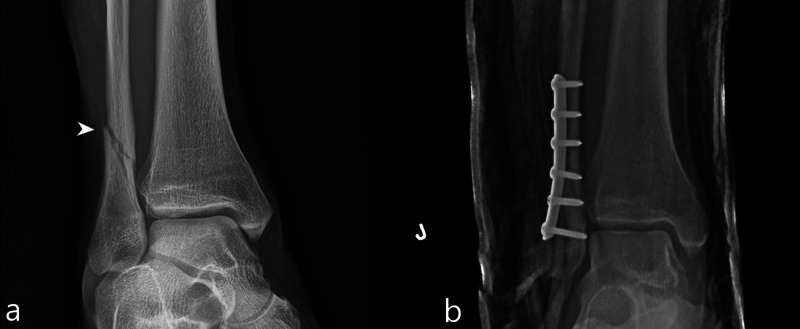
Case 8 radiographic images of the ankle A frontal radiograph of the ankle demonstrates a non-comminuted fracture of the lateral malleolus (white arrowhead). A frontal radiograph of the ankle (b) demonstrates changes related to internal fixation.
